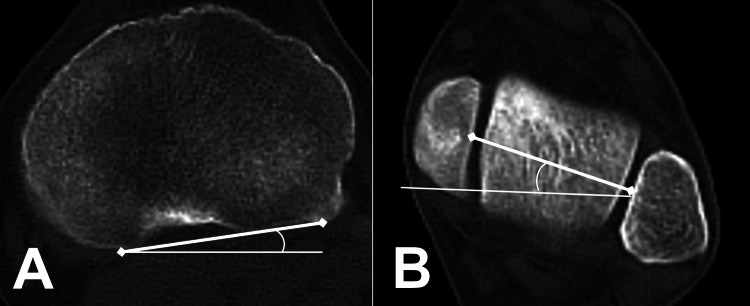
CT measurement of the angle The “tibial posterior condylar axis” was the line connecting the posterior aspects of the lateral and medial condyles at the level just above the fibular head (A). The “bimalleolar axis” was the line connecting the centers of the articular surface between the medial and lateral malleoli at a level just below the tibial plafond (B). “CT tibial external rotation” was the angle measured between the lines of the tibial posterior condylar axis and the bimalleolar axis (angle of (B) - angle of (A)). External rotation was a positive value, and internal rotation was a negative value.
